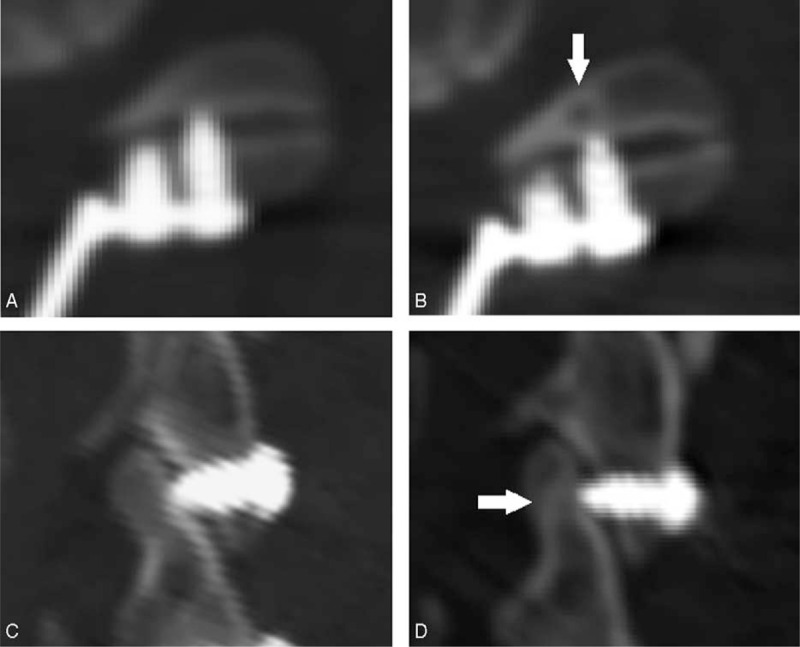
Facet joint osteoarthritis after the facet joint destroyed. (A, B) The axial CT images. (C, D) The sagittal CT images. (A, C) At 1 week postoperative, the facet joint was destroyed by the miniscrew and no osteoarthritis existed. (B, D) Two years follow-up, the facet joint osteoarthritis formed, osteolysis and osteosclerosis existed (the arrow).
